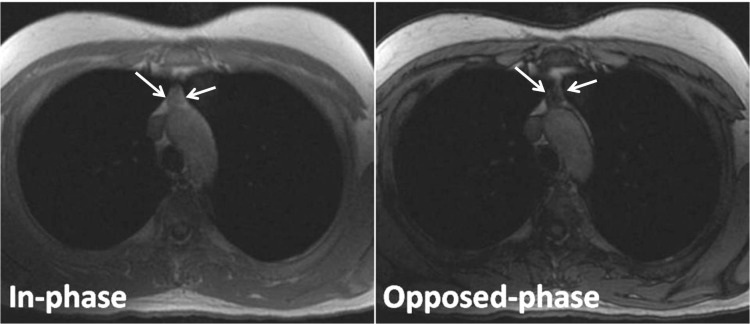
Thymic hyperplasia in a 26-year-old man with myasthenia gravis. Transverse in-phase (155/4.6) and opposed-phase (155/2.1) gradient-echo T1-weighted MR images demonstrate an apparent decrease in signal intensity of the thymus gland on the opposed-phase image relative to the in-phase image (arrows). The CSR is of 0.331.
